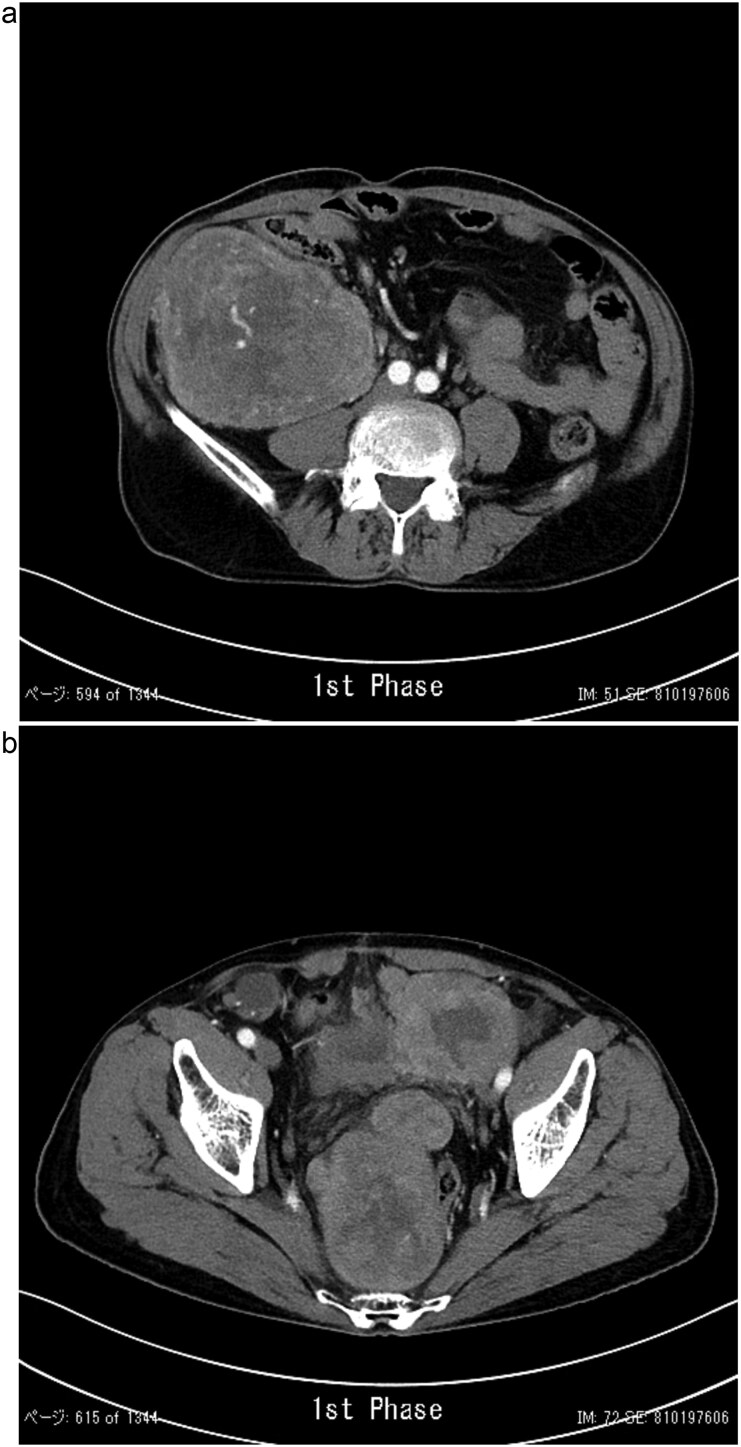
Contrast-enhanced CT showing a lobulated, ill-defined mass with heterogeneous moderate enhancement in the right lower abdomen (a) and several masses occupying the pelvic cavity (b).
